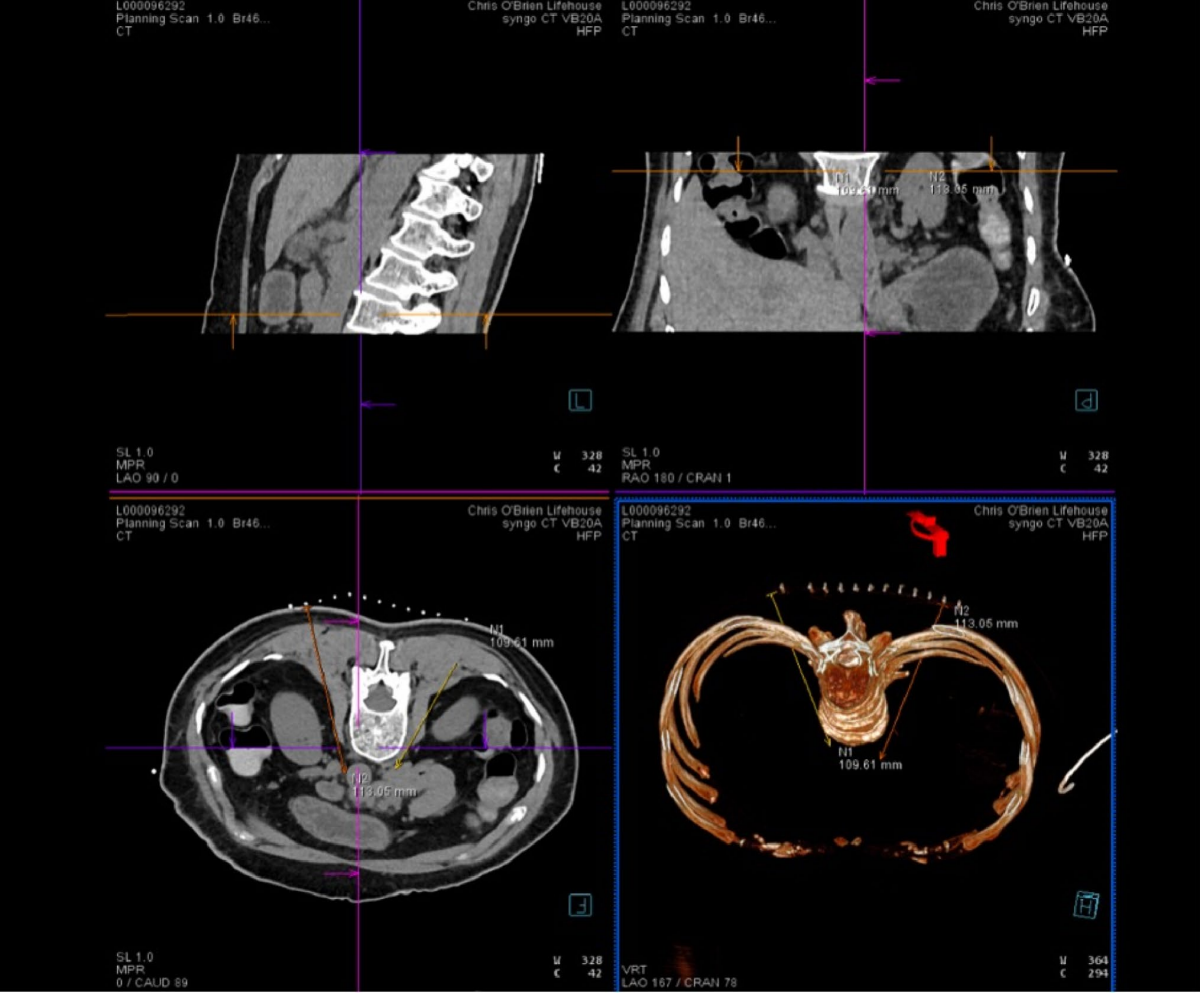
The planning CT scan provides 3-D visualization before needle placement in a patient with advanced pancreatic cancer
